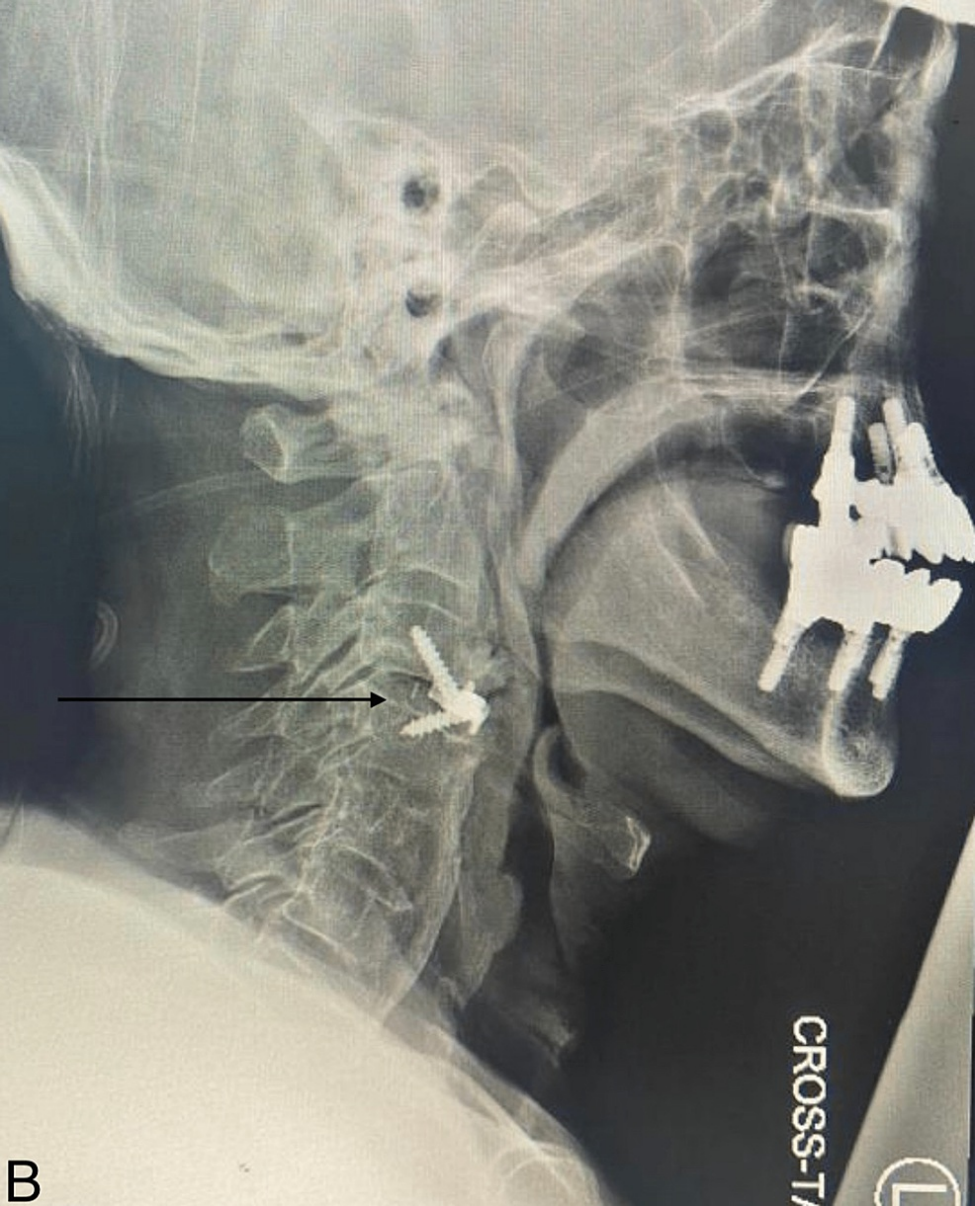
Postoperative X-ray cervical spine; lateral view Showing the removal of osteophytes with fixation of C3-4 by cage and screws
